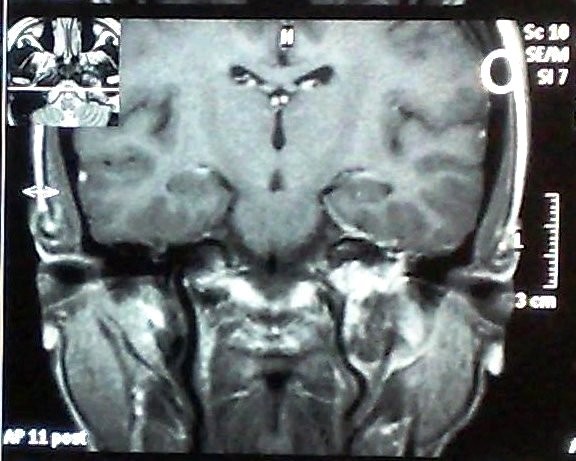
36 months post gamma knife surgery follow-up MRI with contrast for the same case showed loss of central tumor enhancement with reduction of the treated glomus jugulare tumor.
